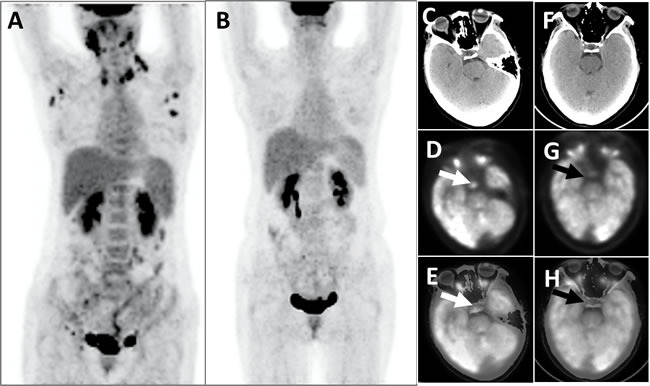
This patient with LCH developed multiple hypermetabolic lymph nodes, which disappeared after chemotherapy The PET whole-body images (MIP), taken prior to chemotherapy, showed that the patients displayed multiple abnormal FDG hypermetabolism (SUVmax = 4.4-13.2) of the lymph nodes in the bilateral submandibular areas, neck, armpits, abdomen, retroperitoneum, pelvic cavity, and bilateral inguinal areas A.; Post-chemotherapy PET whole-body images did not identify aberrant FDG hypermetabolic foci B. Head PET D. and G., CT C. and F. and PET/CT fusion topographic images (E and H) revealed that the pituitary exhibited apparent FDG hypermetabolism (white arrows, SUVmax = 6.0) before chemotherapy and that the metabolic activity was clearly decreased after chemotherapy (black arrows, SUVmax = 3.6).
